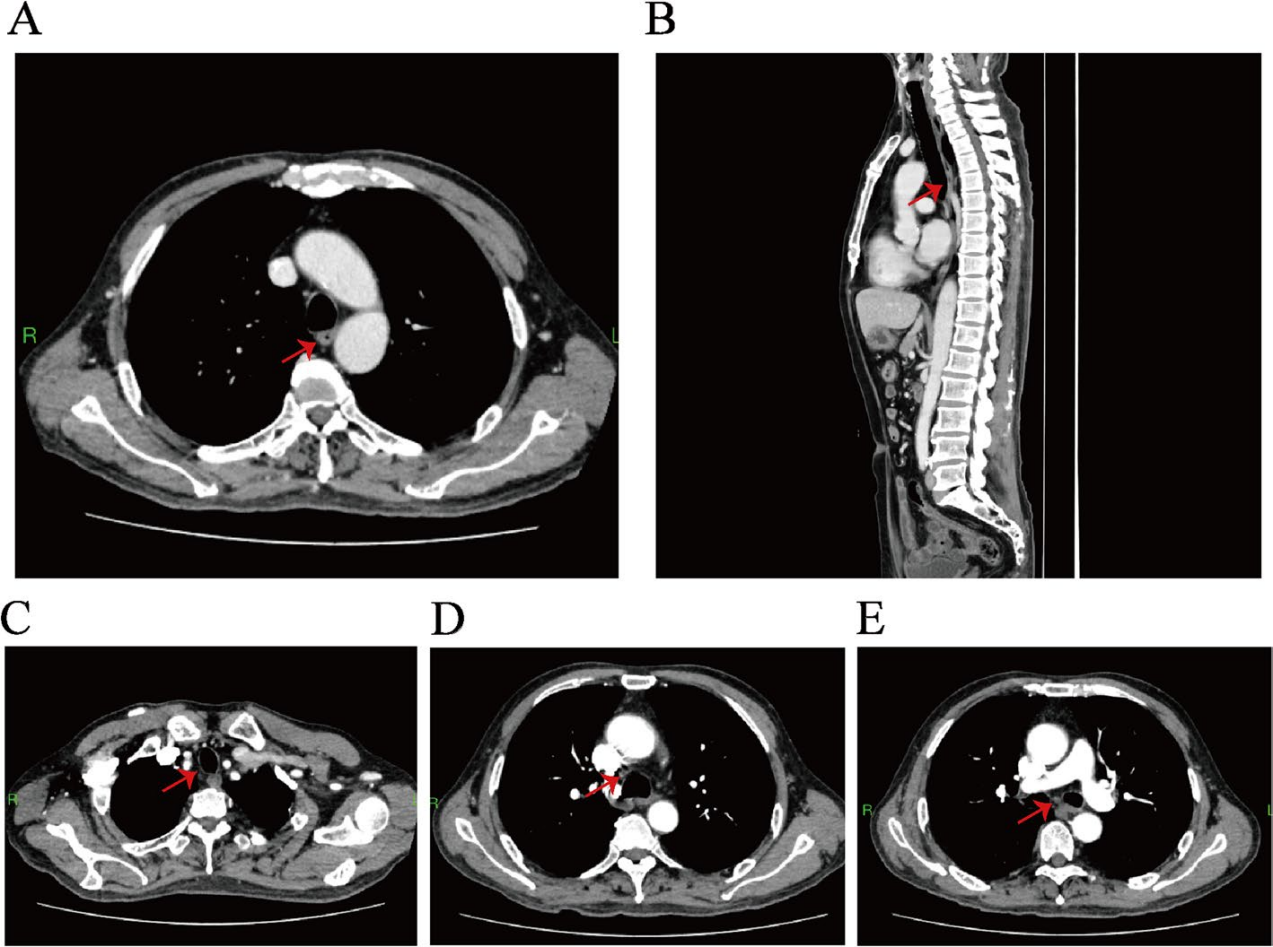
Post-treatment examination: computed tomography (A, B). The red arrows indicate the esophageal cancer lesions. C–E The red arrows indicate lymph nodes. All of them became smaller compared with pre-treatment
